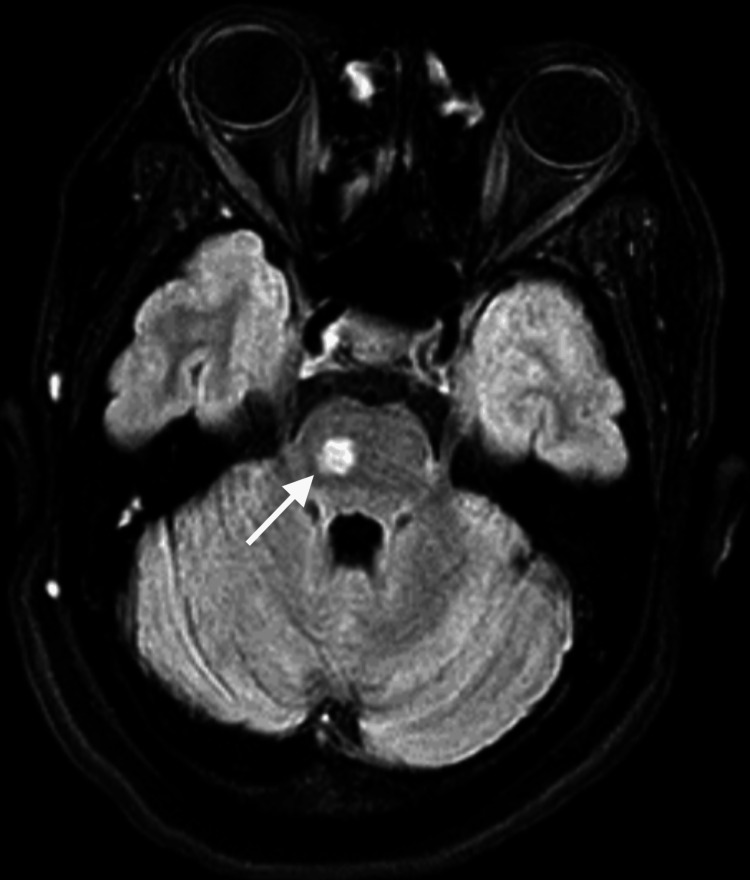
Brain MRI performed after the first seizure (axial section, FLAIR) Right lateral pontine lesion with FLAIR hyperintensity (white arrow) MRI: magnetic resonance imaging; FLAIR: fluid-attenuated inversion recovery
